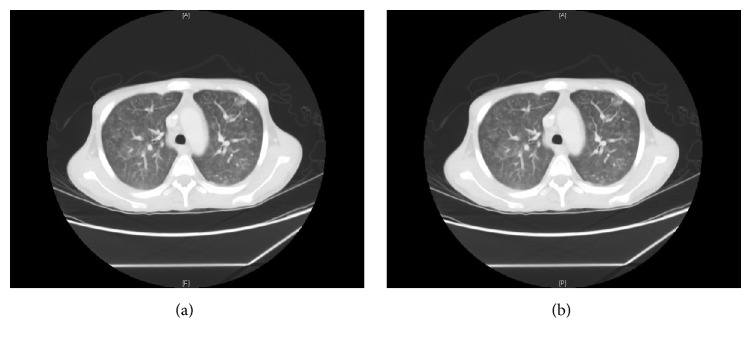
(a) CT scan showing ill-defined centrilobular nodules. (b) CT scans showing ill-defined ground glass density mainly in the upper lobes.
